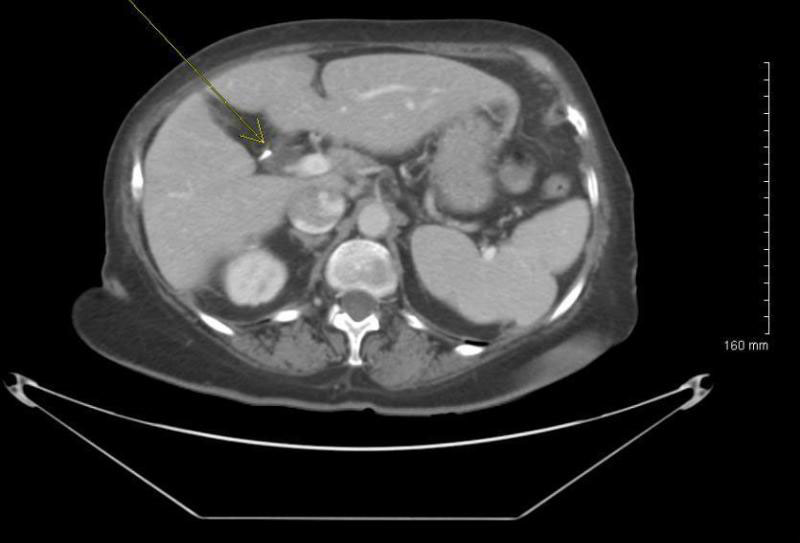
CT scan of the liver demonstrating mass concerning for abscess and tumor.
